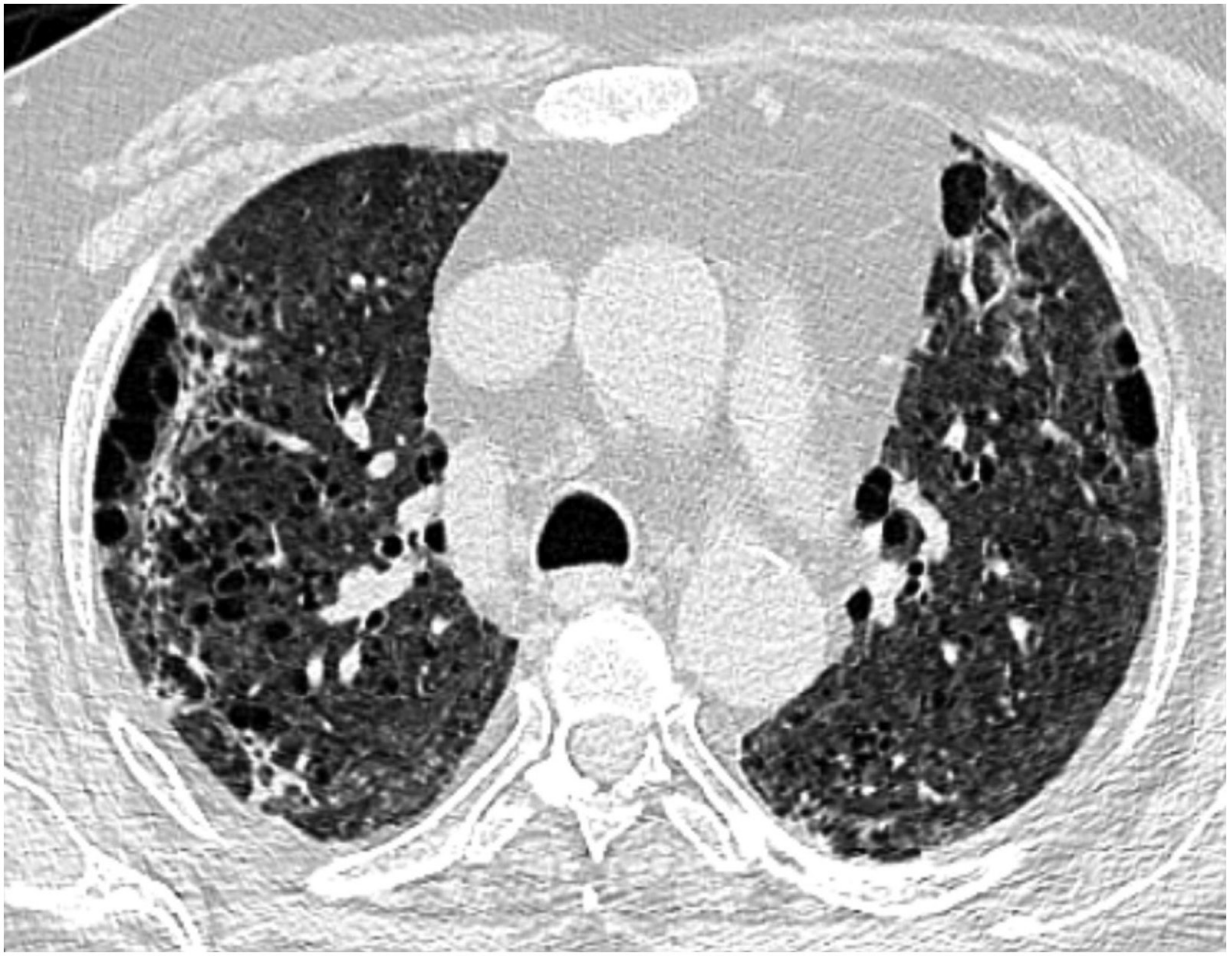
Improvement of central parenchyma while peripheral emphysema was more prominent at the first follow-up HRCT.
